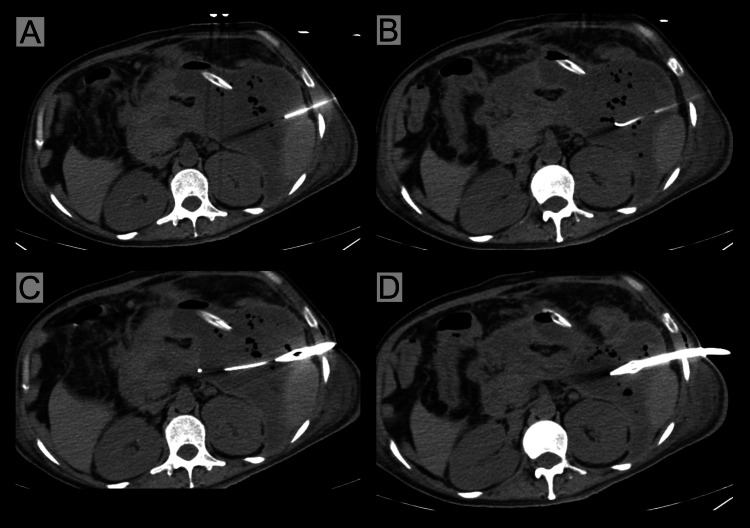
Computed tomography-guided percutaneous drainage of infected pancreatic necrosis. (A) Needle insertion, (B) guidewire insertion, (C) catheter insertion after tract dilatation, and (D) final position of the catheter Image credits: Dr. Swati Das Patients' consent was taken for the publication of the image.
